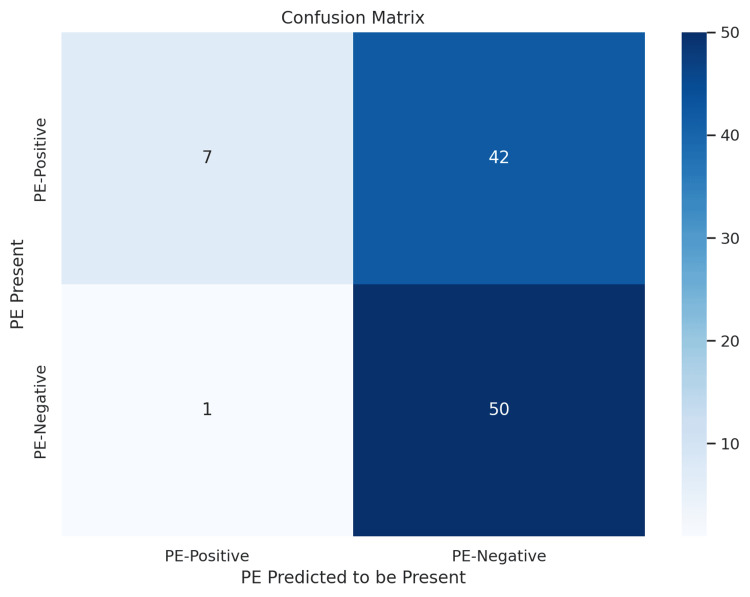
Confusion matrix for Gemini 2.0 model predictions on CTPA images Confusion matrix displaying Gemini 2.0 model performance in identifying pulmonary embolism (PE) from CTPA images. Rows represent the actual presence or absence of PE, while columns indicate the model’s predicted classification. The model correctly identified 50 of 51 PE-negative cases and seven of 49 PE-positive cases. This matrix demonstrates Gemini 2.0’s strong performance in ruling out PE but lower sensitivity in detecting PE presence CTPA: computed tomography pulmonary angiography
